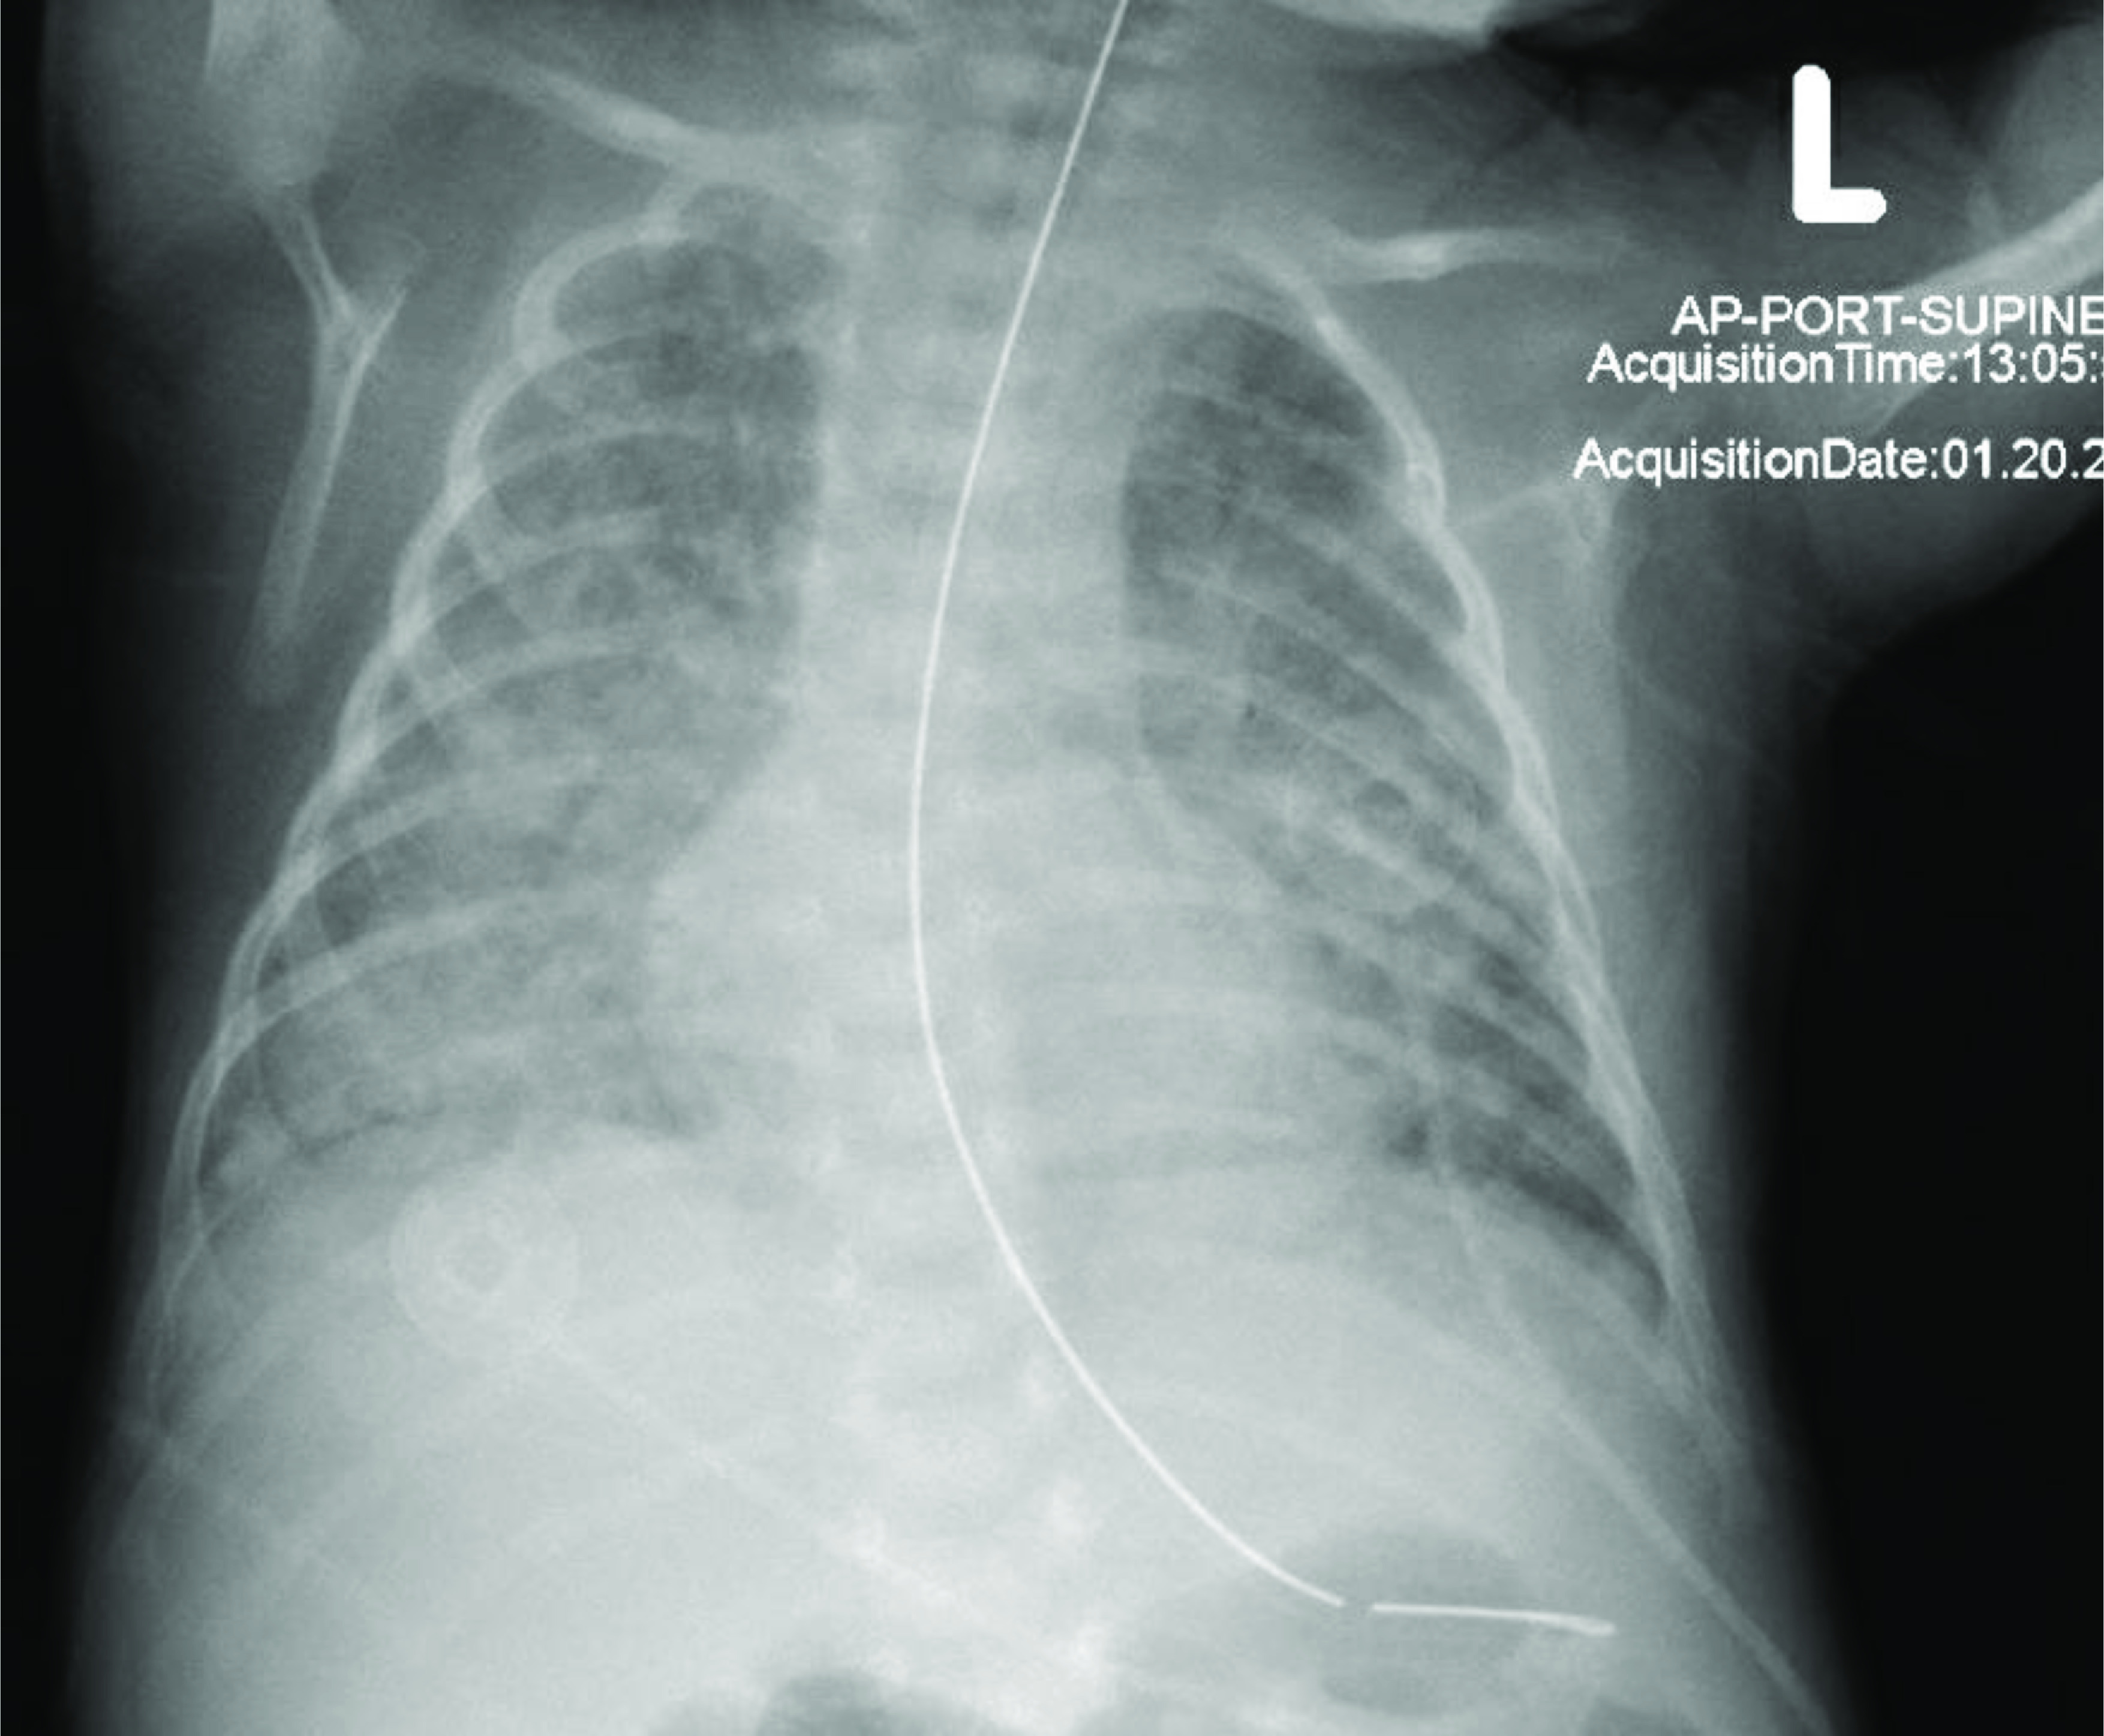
Chest X-ray with diffuse alveolar and interstitial opacities, *R* > *L*, possibly representative of developing pneumonia/ARDS.
